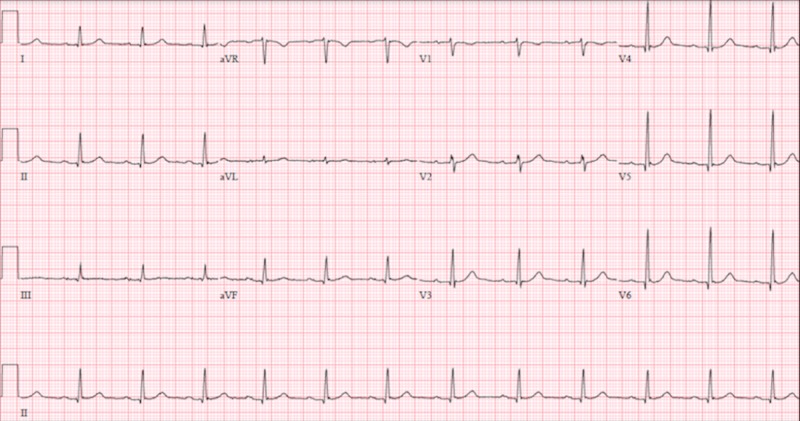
12-lead echocardiogram post nitroglycering administration with resolution of inferolateral ST-elevations
